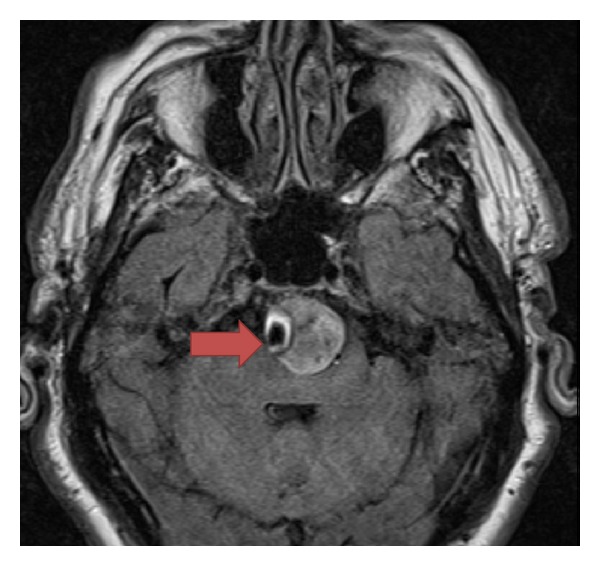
MRI Interpretation: Large partially thrombosed 25 mm basilar artery aneurysm without evidence of rupture. There is an acute or early subacute infarct within the left posterior inferior cerebellar artery distribution. There is also a questionable small focus of acute or early subacute infarct at the left posterolateral pontomedullary junction. There is no hydrocephalus or herniation.
